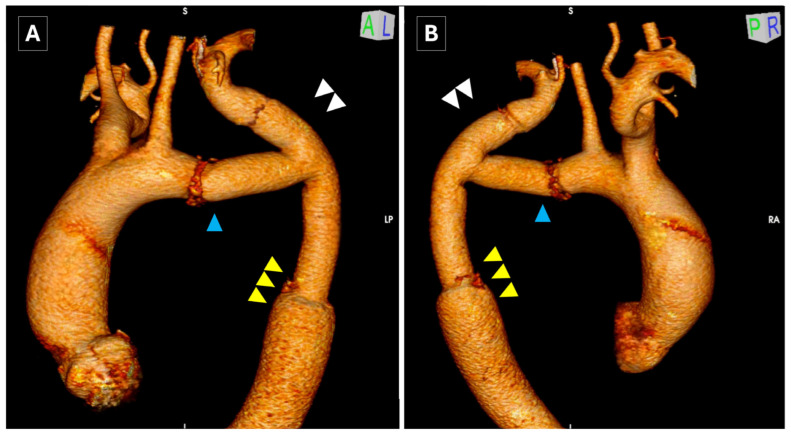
Three-dimensional CTA reconstruction at 6 months ((A): anterior view; (B): posterior view), showing the distal aortic arch reconstruction (blue arrowhead), the patency of the LSA (white double arrowhead) and the distal thoracic aorta (yellow triple arrow).
